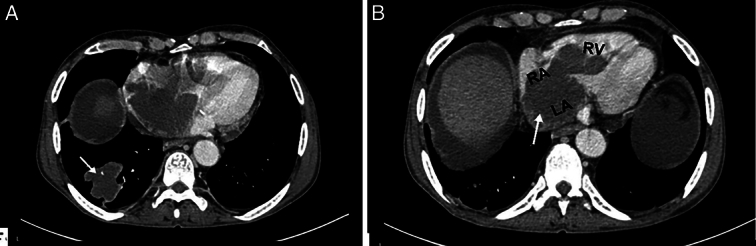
Contrast-enhanced computed tomography of the chest demonstrates right primary lung malignancy [white arrow in (A)], and IVC thrombus extending into the left atrium (LA), right atrium (RA), and right ventricle (RV) [white arrow in (B)].
